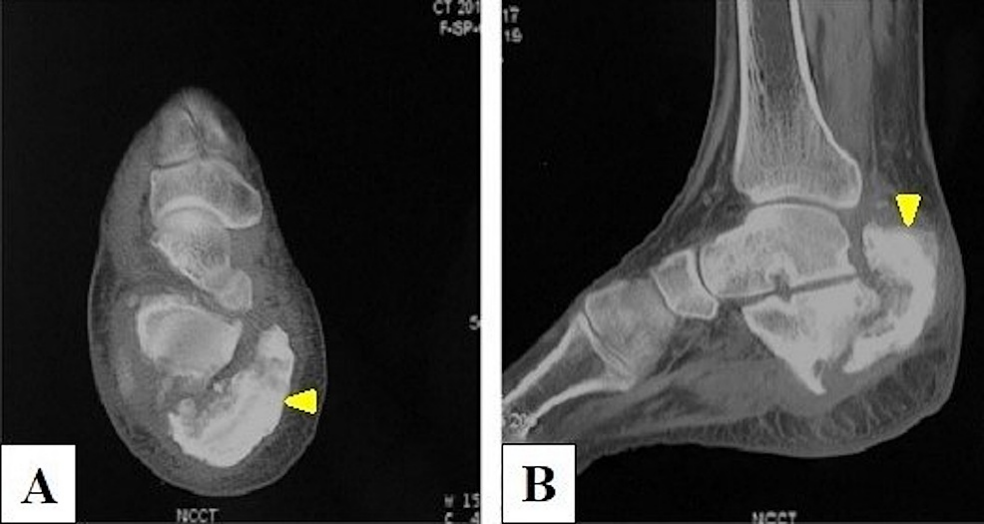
Computed tomography of the heel region. (A) Axial view showing the displaced posteromedial fracture fragment bearing the whole of the calcaneal tuberosity (yellow arrowhead). (B) Lateral view showing posterosuperior displacement of tuberosity fragment (yellow arrowhead).
